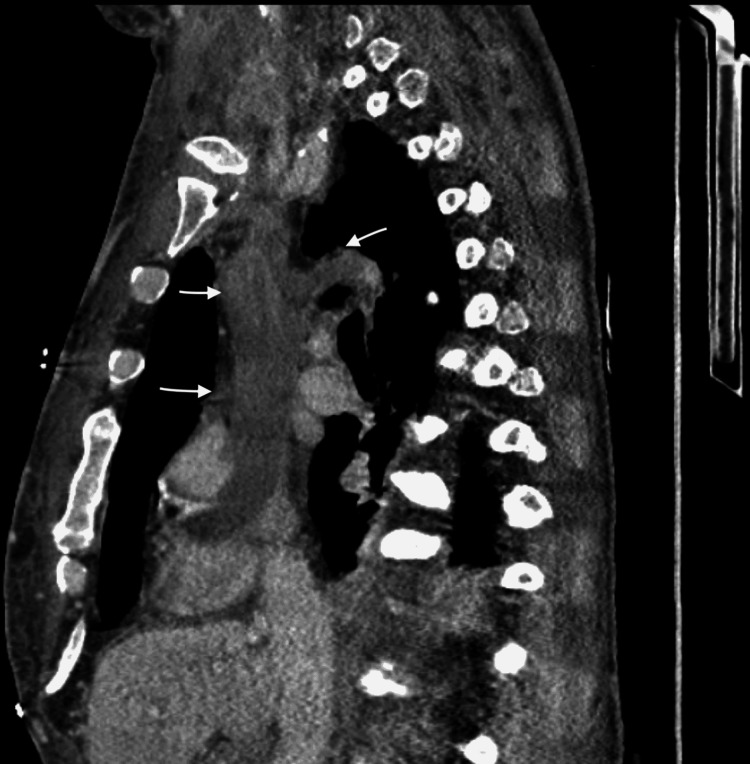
Sagittal chest CT scan with arrows showing thrombus in SVC and azygos vein
